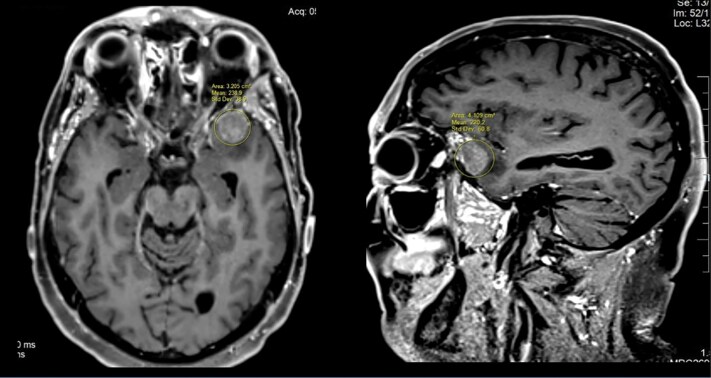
Purely dural-based metastasis. Axial and sagittal T1-weighted MR images showing an enhancing dural-based mass in the anterior left middle cranial fossa. The lesion does not involve the overlying skull.
